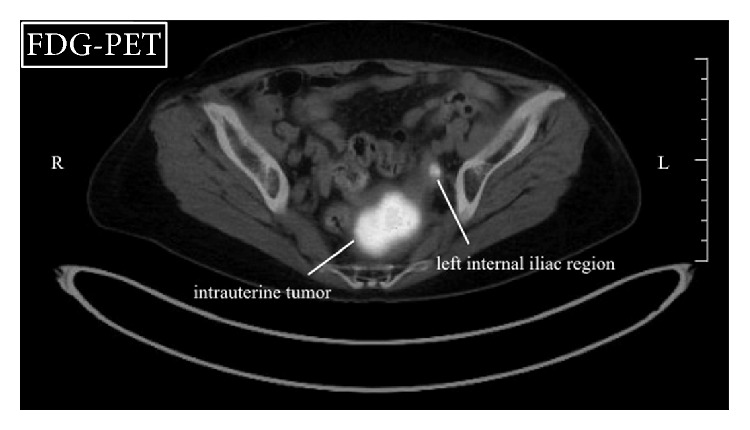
Findings of FDG-PET. Intense accumulation of FDG is observed not only in the uterine tumor but also in the left internal iliac region.
